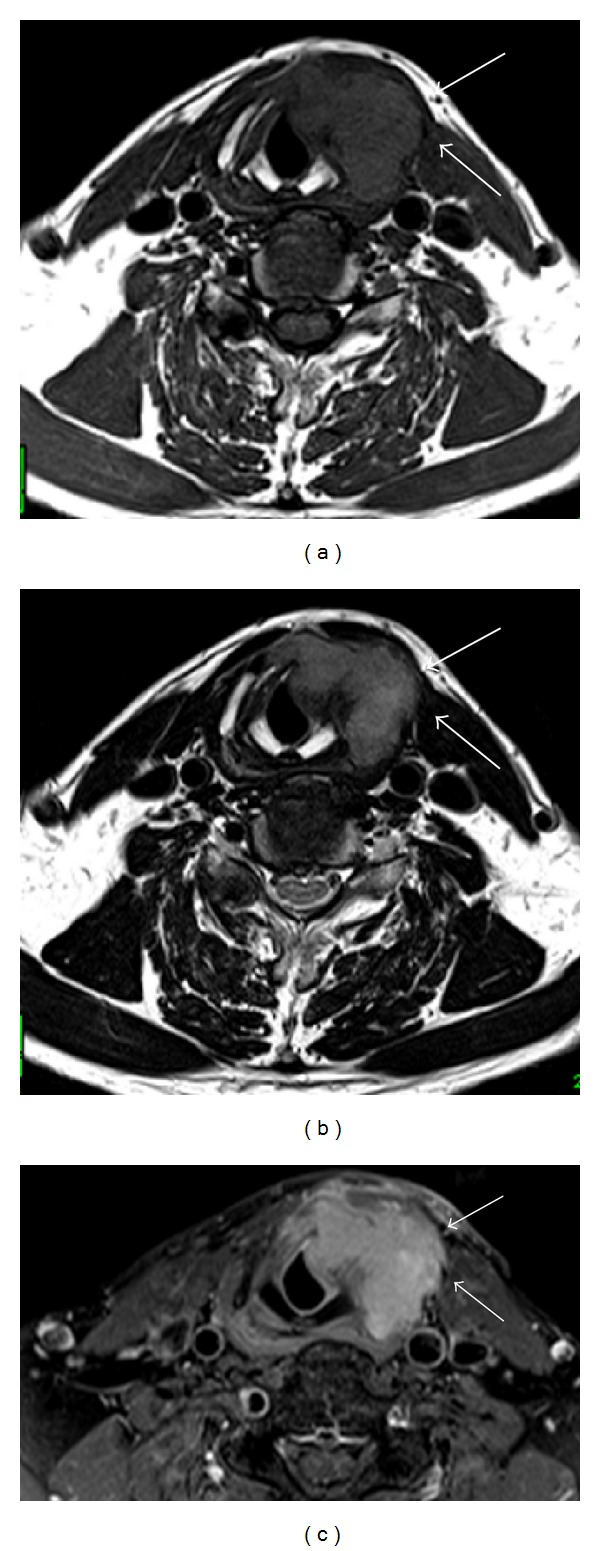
MRI revealed the same findings as CT scanning: (a) a low-intensity area on T1-weighted images and (b) a nonhomogenous high-intensity area on T2-weighted images. (c) The tumor demonstrates high intensity on a postgadolinium contrast T1 image (arrow).
